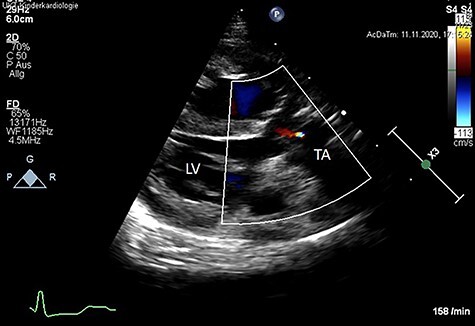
Post-operative color Doppler echocardiography (parasternal long axis view) reveals a competent TV with just a minor trace of central regurgitation. Ao: Aorta, LV: left ventricle, TA: truncus arteriosus.
